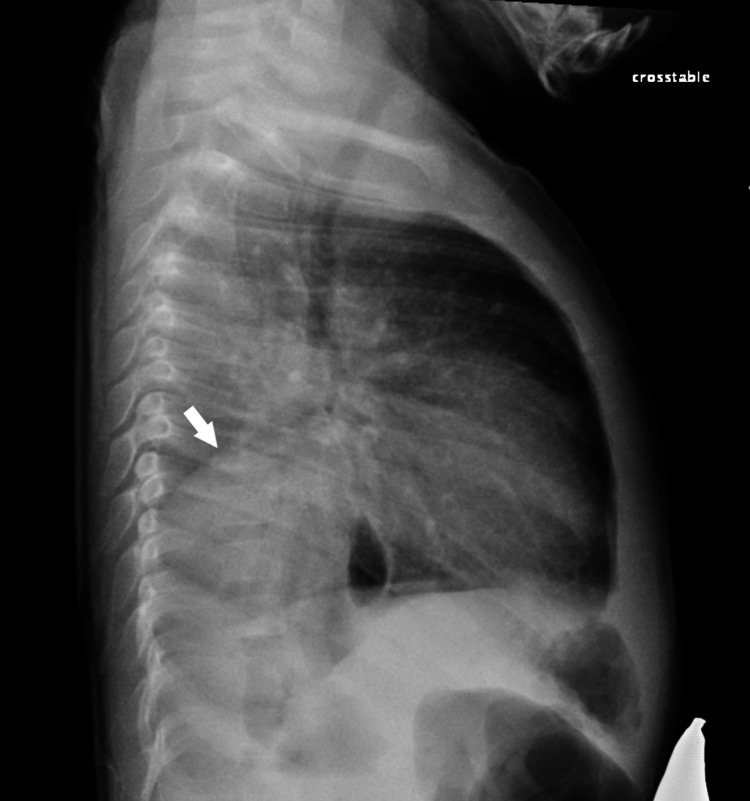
Lateral chest radiography Lateral chest X-ray showing opacity in the lower lobes posteriorly (arrow).
